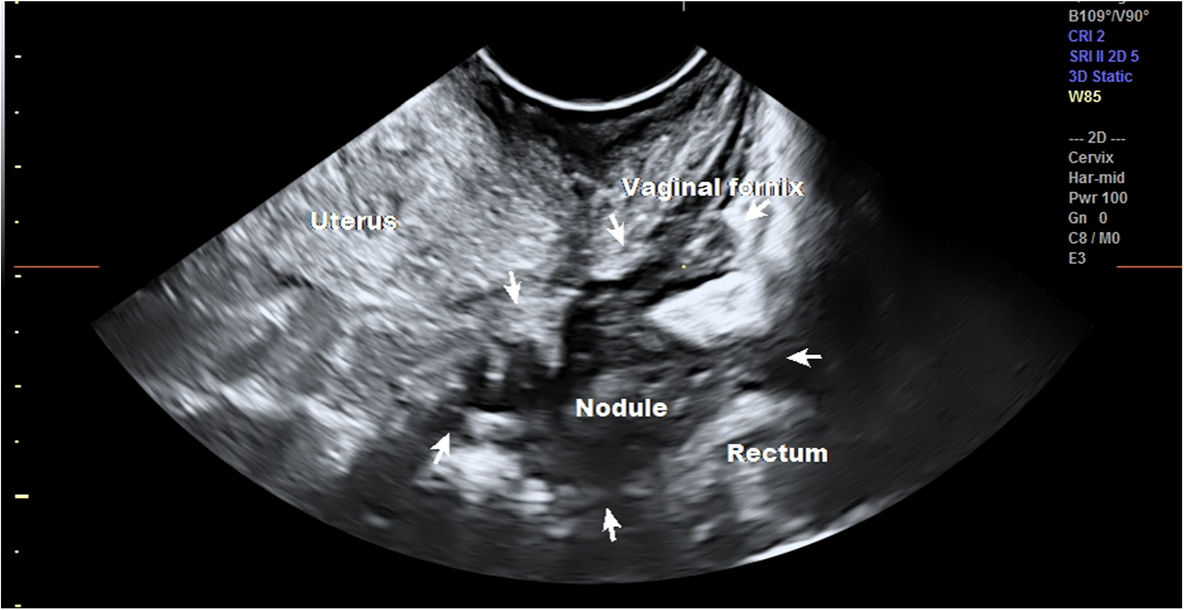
TVUS of a large vaginal nodule extending to the rectosigmoid. The sonographer interpreted this lesion as a rectosigmoid bowel lesion while the surgeon described it as a vaginal lesion. Arrows show extent of lesion
